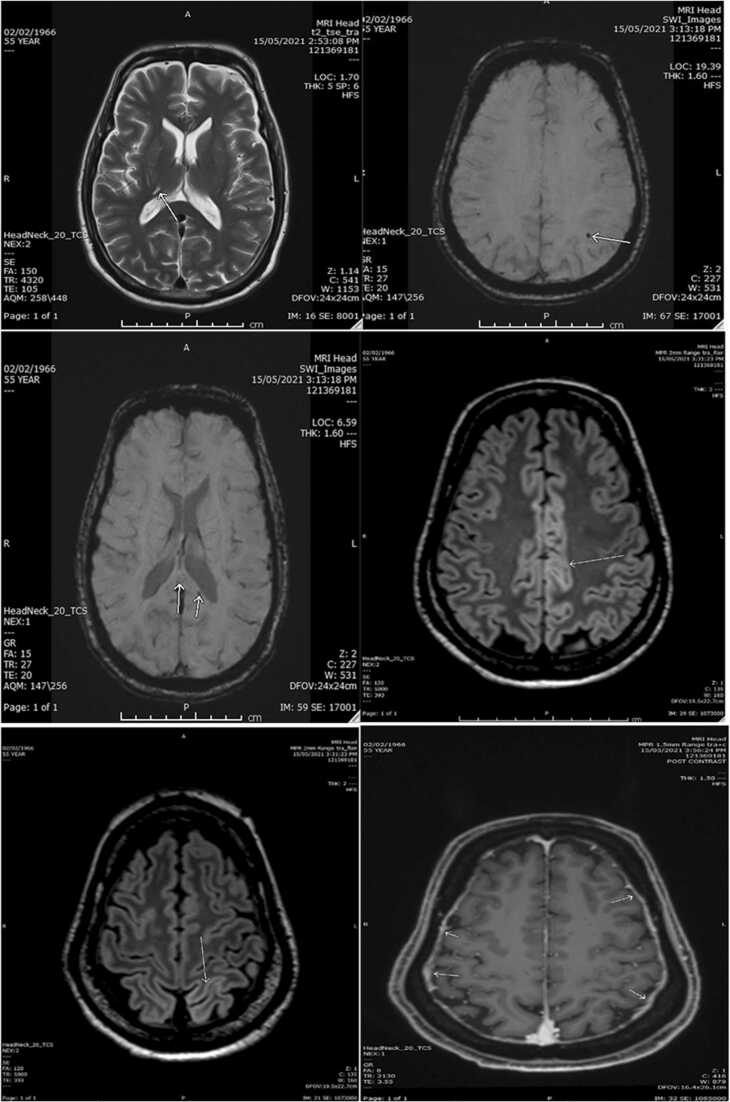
MRI Brain; Leptomeningeal Enhancement and Encephalopathy features.
